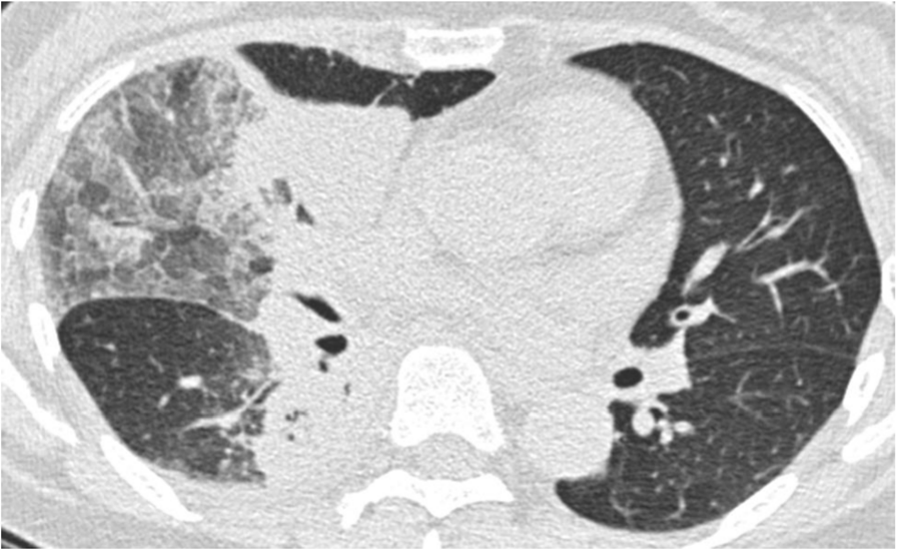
Computed tomography image of the chest showing infiltration in the right middle and lower lobes
